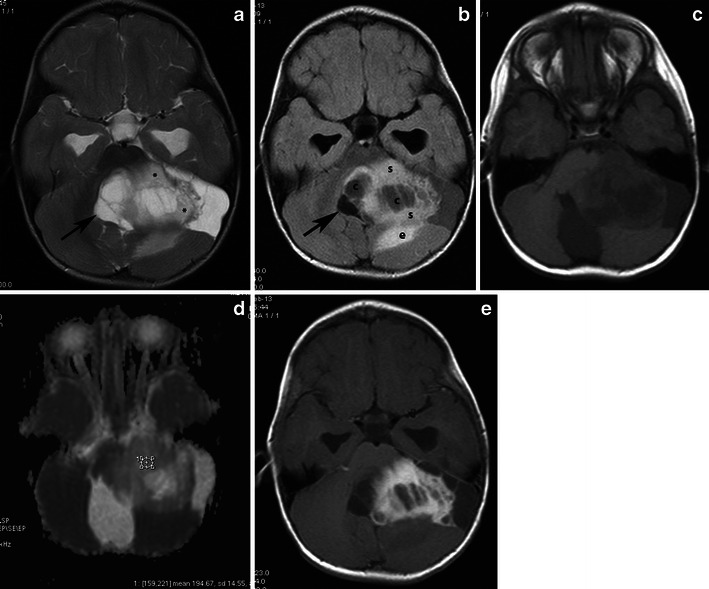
Pilocytic astrocytoma of the left cerebellar hemisphere with a solid and cystic component severely compressing the fourth ventricle and the medulla, producing obstructive hydrocephalus. a An axial T2-weighted image shows a cystic, hyperintense mass with a less intense solid component (asterisks) compressing the fourth ventricle (arrow). b On a FLAIR image, the cystic component (c) shows low signal intensity, which is higher than that of the CSF, and the solid component (s) shows high signal intensity. Note the low signal of the compressed fourth ventricle (arrow) and the peritumoral oedema (e). c On this T1-weighted image, the solid components appear hypointense compared with grey matter. d The solid component shows high values on an ADC map. e Intense enhancement of solid components is depicted after the administration of a paramagnetic agent
